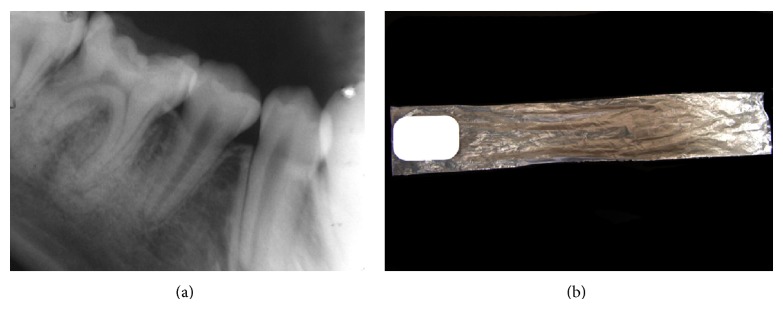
Periapical radiographic examination showing the presence of the first permanent molar with extensive caries and periodontal ligament thickening (a). An intraoral radiographic film enclosed in a plastic bag (4 × 23 cm) (b).
